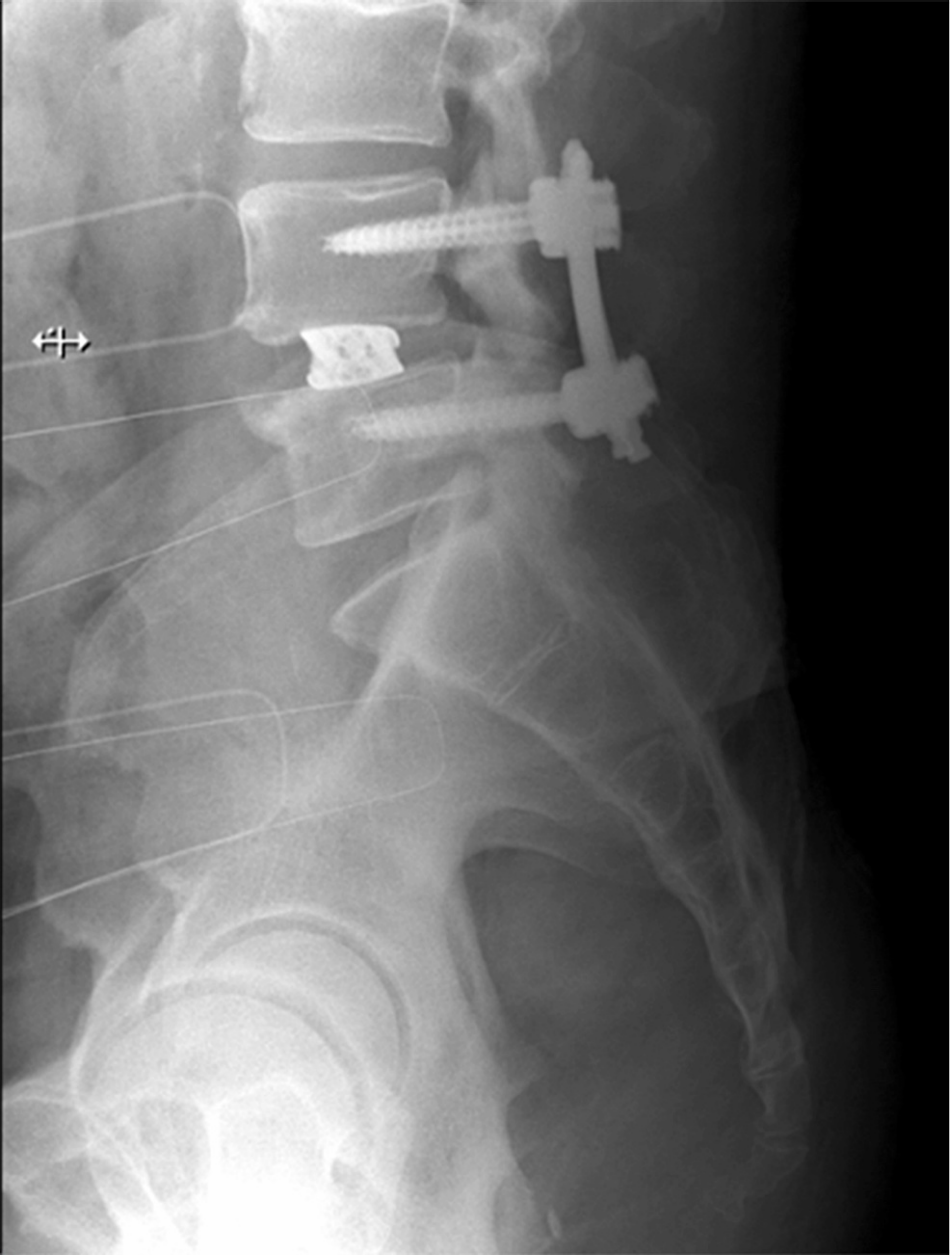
Post-operative lateral lumbar spine X-ray showing successful placement of instrumentation after L4-L5 lateral interbody fusion with endoscopic ipsi-contra decompression, indicating proper alignment and stability with no signs of complication.
